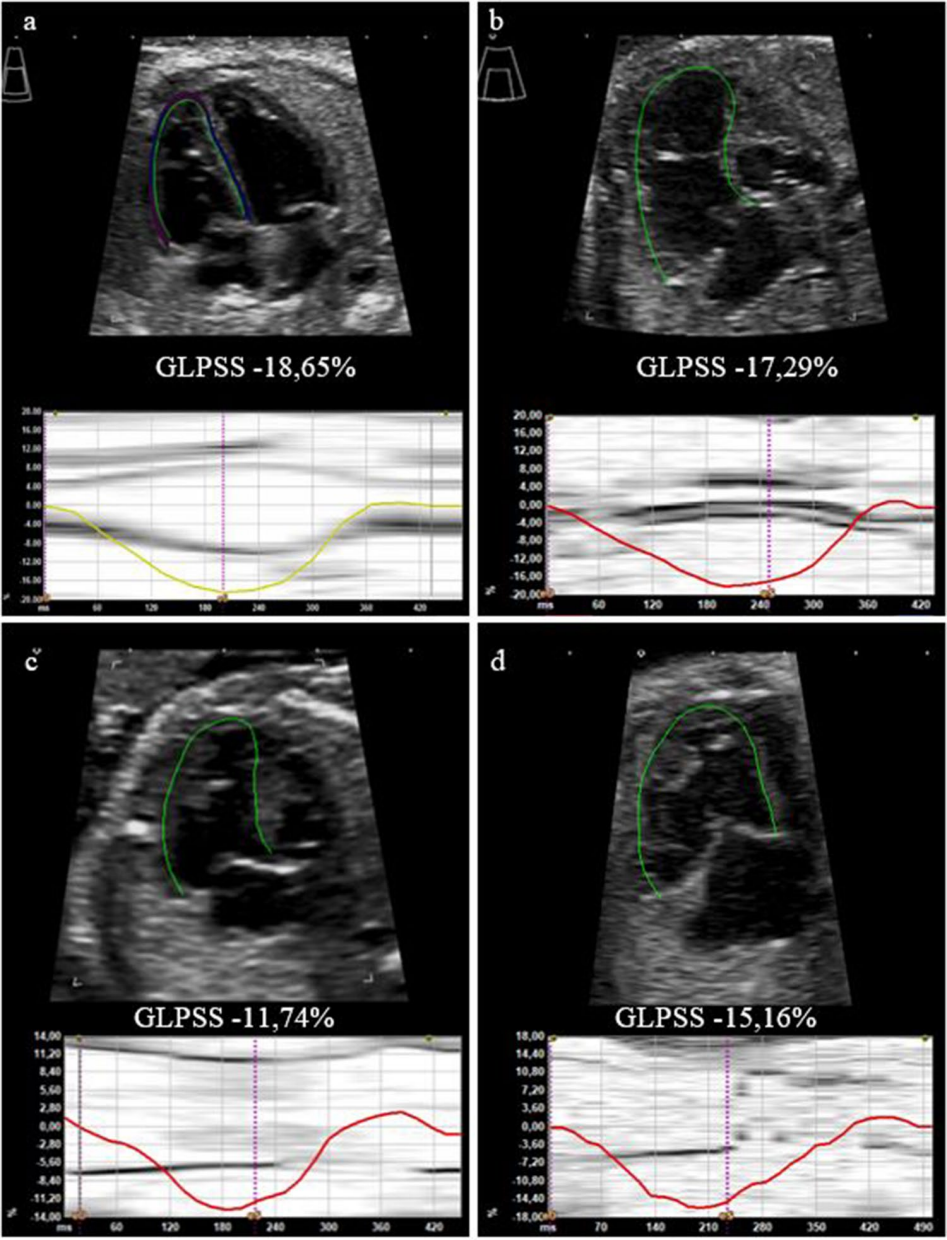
a, b Traced myocardial wall of the right ventricle (B-mode sonography) and global longitudinal strain (%) curves for one fetal heart cycle for a healthy fetus (a) and a fetus with hypoplastic left heart (HLH, MA, AA) at 22 + 1 weeks (b). c, d Traced myocardial wall of the right ventricle (B-mode-sonography) and global longitudinal strain (%) curves for one fetal heart cycle for a fetus with HLH (MS, AA) and EFE (c) at 21 + 6 weeks and for a fetus with HLH (MS, AS) without EFE (d) at 27 + 3 weeks. There is a tendency for lower GLPSS values in the case of HLH, especially for additionally existing EFE. *AA, aortic atresia; AS, aortic stenosis; EFE, endocardial fibroelastosis; MA, mitral atresia; MS, mitral stenosis
